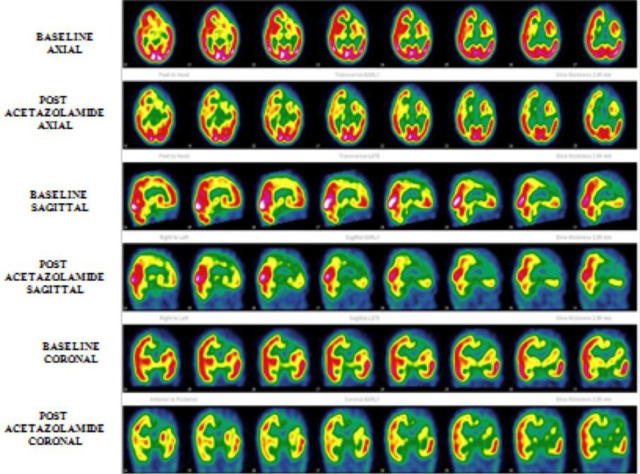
Baseline and post-ACZ SPECT images in an 11-year-old male with recurrent seizures and right hemiparesis show paradoxical perfusion worsening in the left frontal region and other regions post-ACZ, consistent with a Type III response indicating exhausted cerebrovascular reserve
